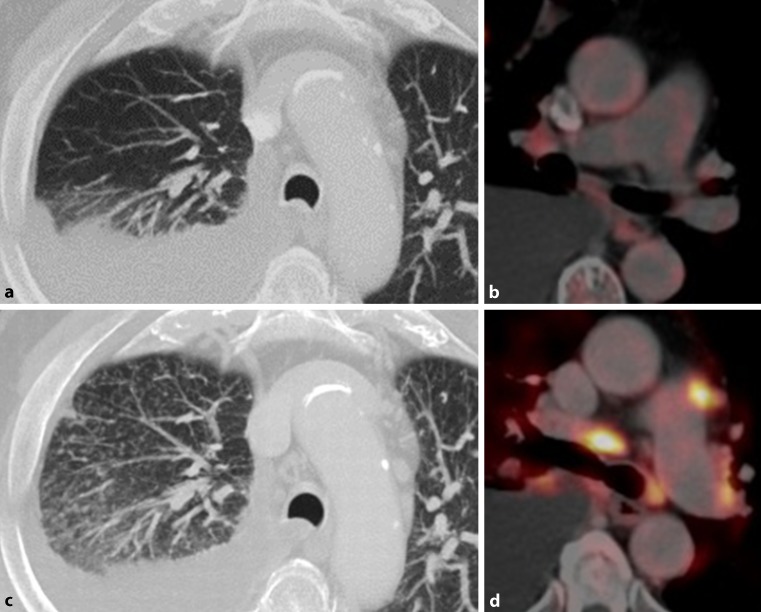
Sarcoid-like reaction in a 77-year-old man with non-small cell lung cancer receiving immune checkpoint inhibitor therapy. a, b Axial fluorodeoxyglucose positron emission tomography/computed tomography (^18^F-FDG-PET/CT) image obtained before therapy demonstrate a malignant right pleural effusion. c, d 4 weeks after treatment initiation, numerous intrapulmonary micronodules were detectable in both lungs, predominately right-sided. In addition, enlarged hilar/mediastinal lymph nodes with increased ^18^F-FDG newly developed, consistent with a sarcoid-like reaction. In contrast, malignant tumor burden resolved completely
